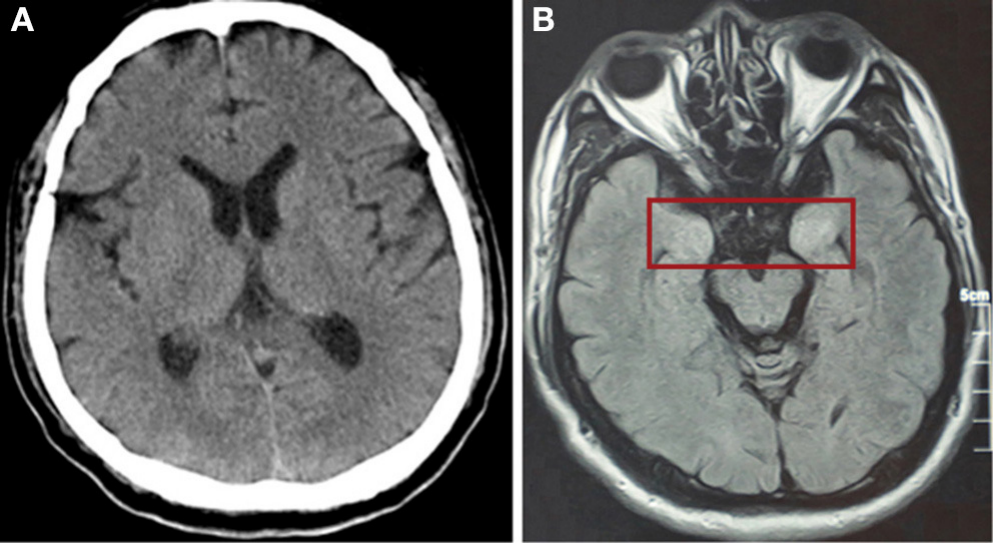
(A) CT scan of the brain showed no abnormally high or low opacity on 31st January (day 14 of the illness). (B) Magnetic resonance scan of the brain after discharge showed abnormalities (in red box) in the bilateral temporal lobe and hippocampus on 5th May (day 81 of the illness).
